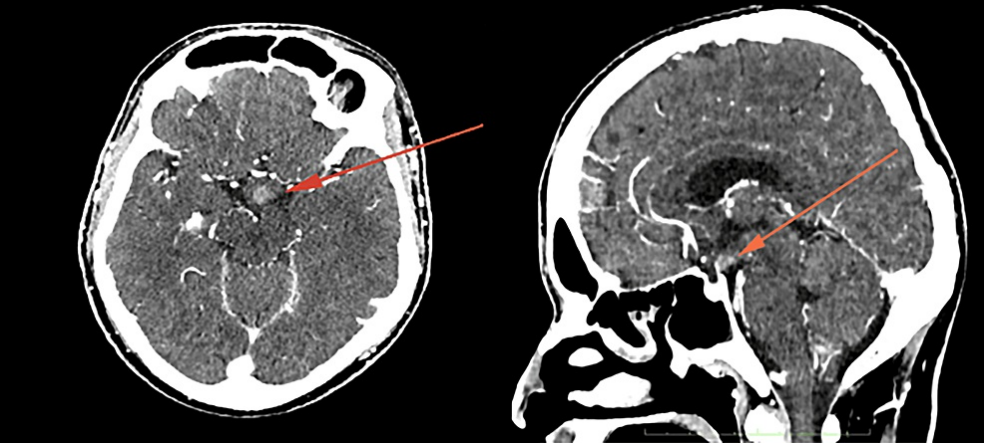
Enhancing hypothalamic lesion noted in the same contrasted CT brain (arrow) along with the left periventricular rim enhancing lesion.
